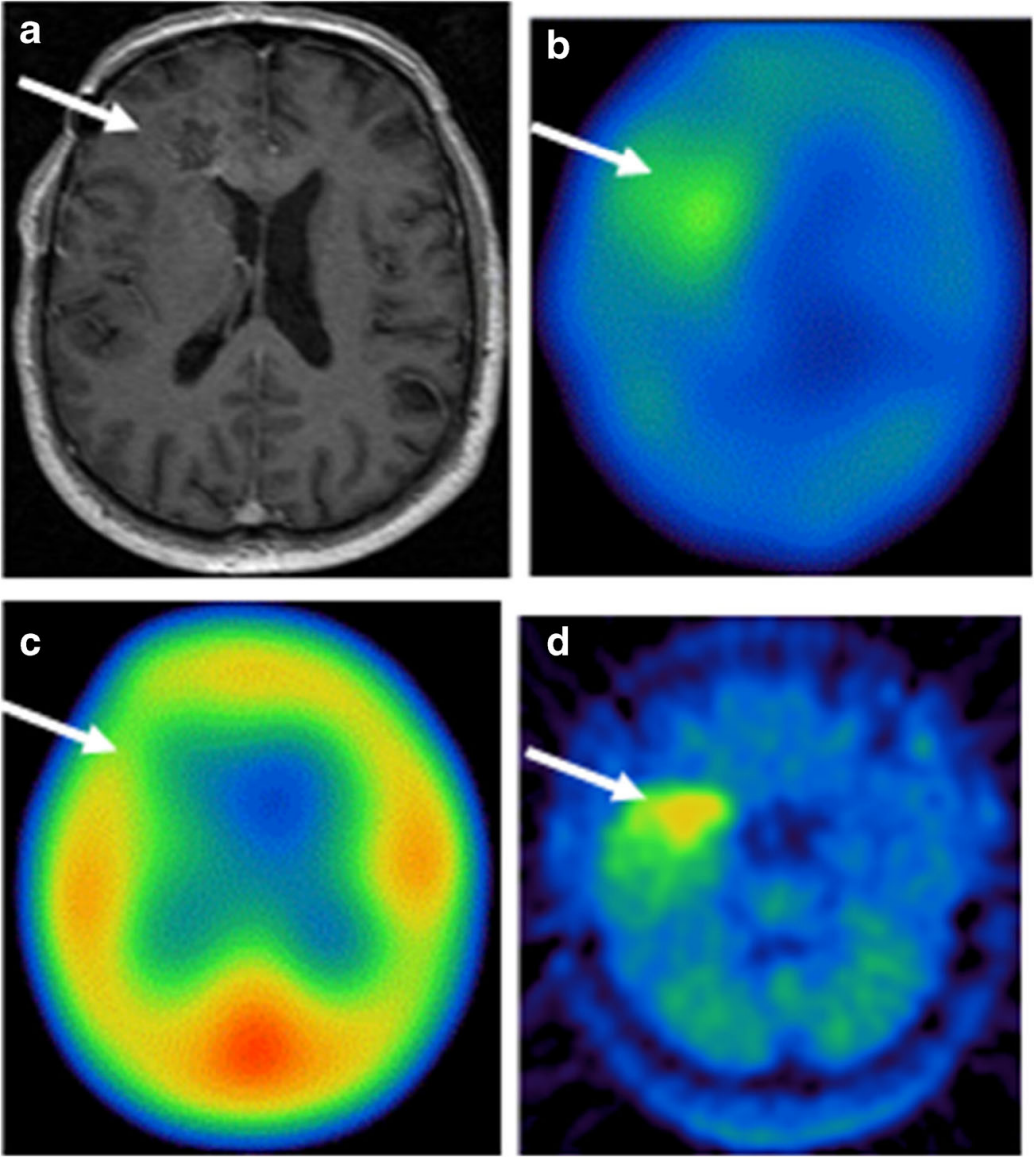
A 66-year-old man with grade IV glioma in the right frontal lobe. Axial T1-weighted MRI showed the lesion in the right frontal lobe with enhancement (a). The tumor lesion was positive in [^123^I]-VEGF SPECT imaging 18 h after injection of [^123^I]-VEGF (b), and no significant [^123^I]-VEGF accumulation was demonstrated 30 min after the [^123^I]-VEGF application (c). The lesion was positive in [^11^C]-MET PET (d). White arrows indicate the tumor region
